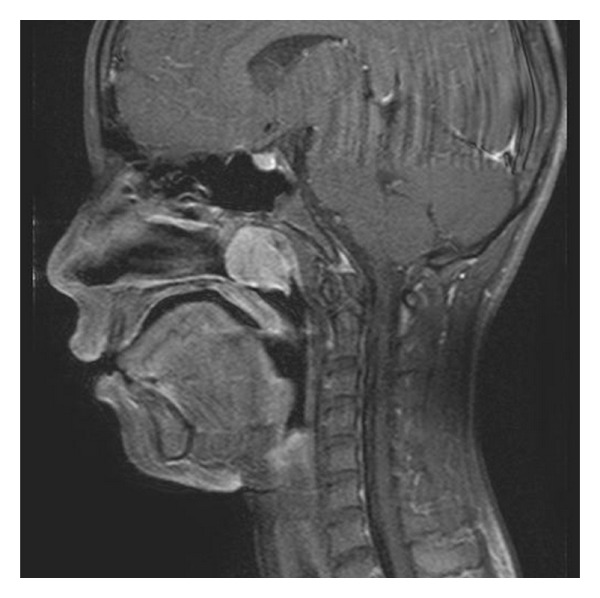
Sagittal T1-weighted magnetic resonance image with gadolinium showing a soft tissue mass with homogeneous enhancement.
